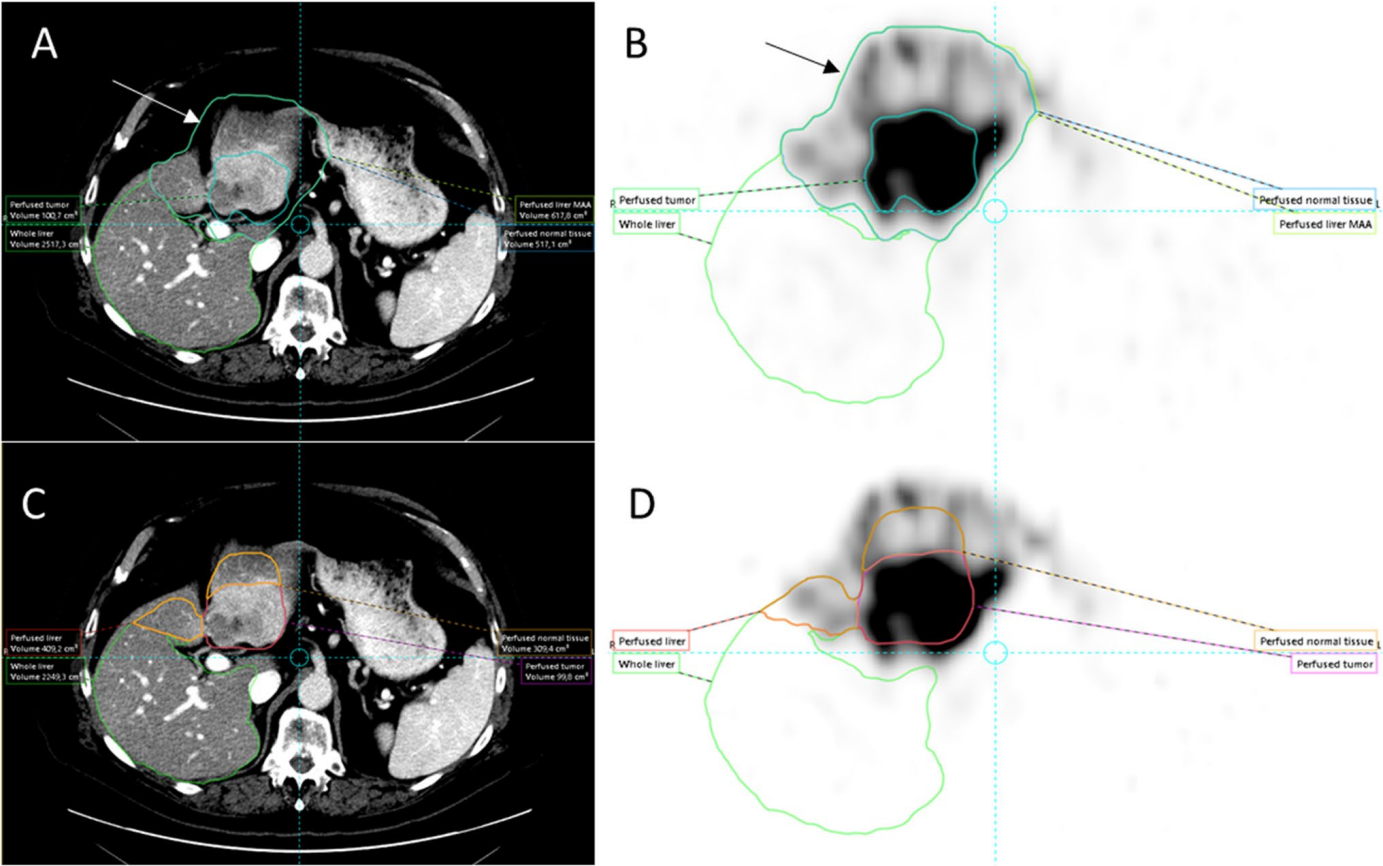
Threshold-based segmentation of the tumour on SPECT (panels A and B) is similar to CT-based segmentation (panels C and D) because of well-defined.^99m^Tc-MAA accumulation in the tumour and contrast enhancement on CT. Both methods work in this case. Note: perfused volume definition between both methods is very different with overestimation on SPECT (arrows)
